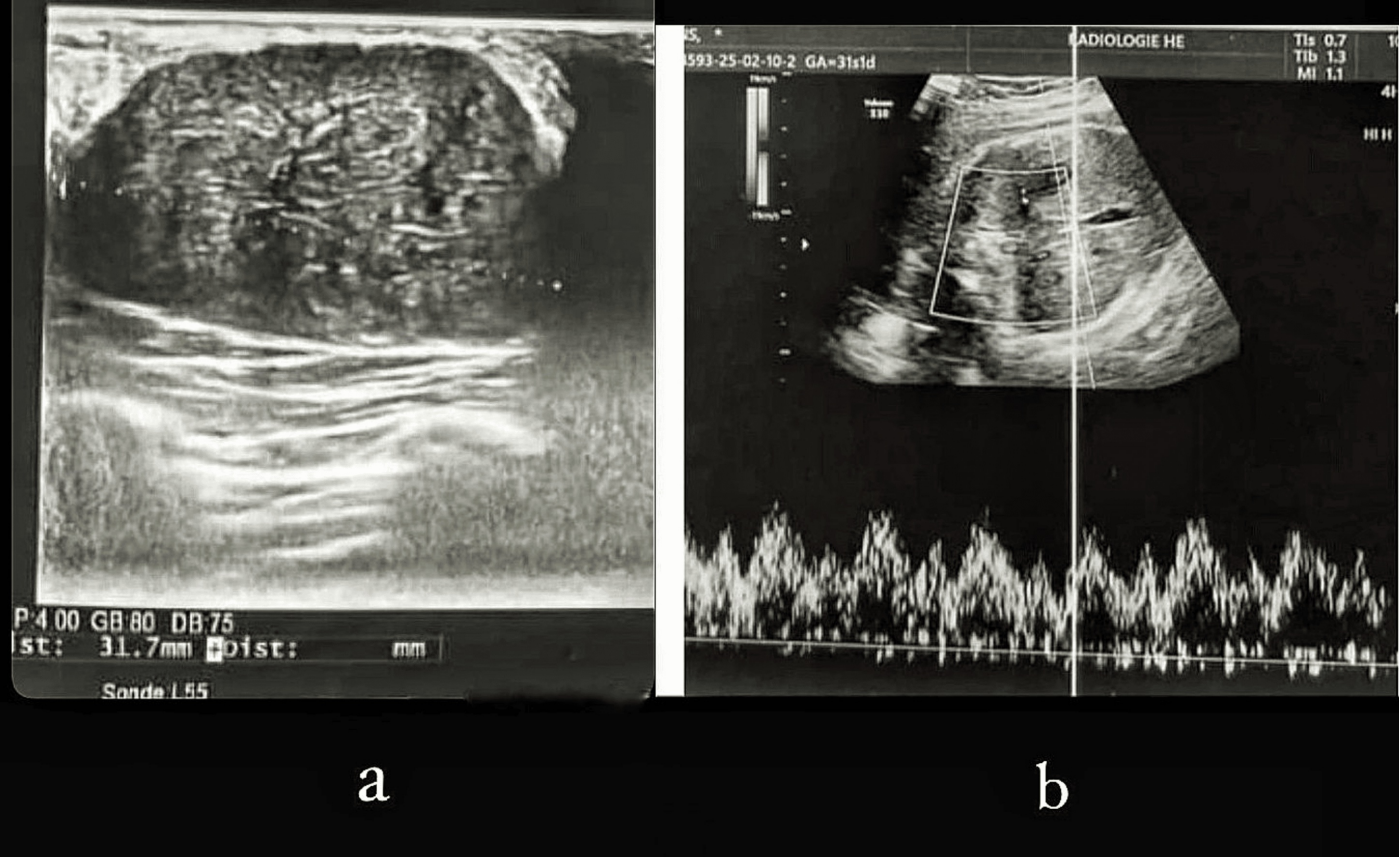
Breast ultrasound images of the present case (a) B-mode ultrasound image showing the mass. (b) Pulsed Doppler ultrasound image showing central vascularization of the mass.
